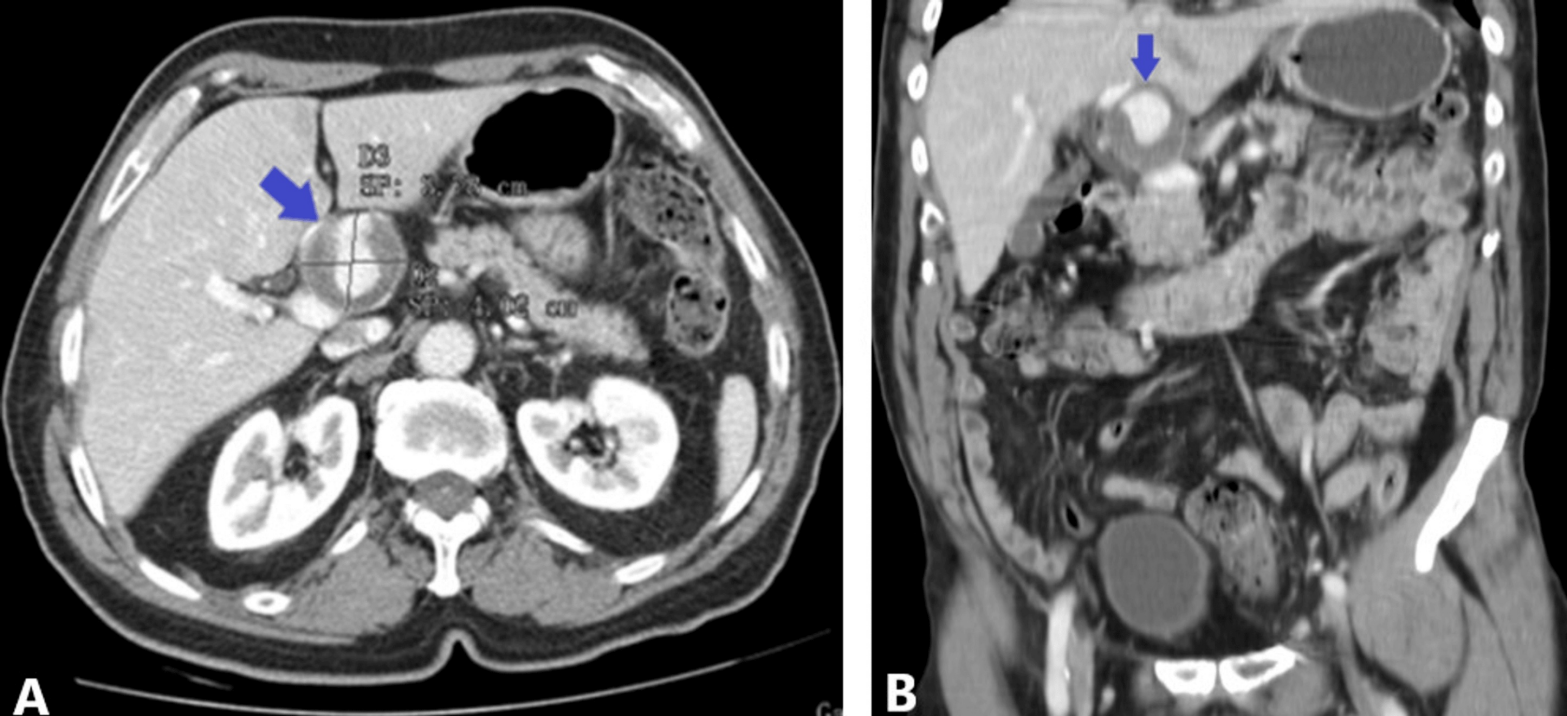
Pretreatment contrast-enhanced multidetector CT of hepatic artery aneurysm CT: computed tomography Pretreatment contrast-enhanced multidetector CT demonstrating a large hepatic artery aneurysm with partial mural thrombosis measuring 37 mm in maximum diameter. (A) Axial view (arrow). (B) Coronal view (arrow)
